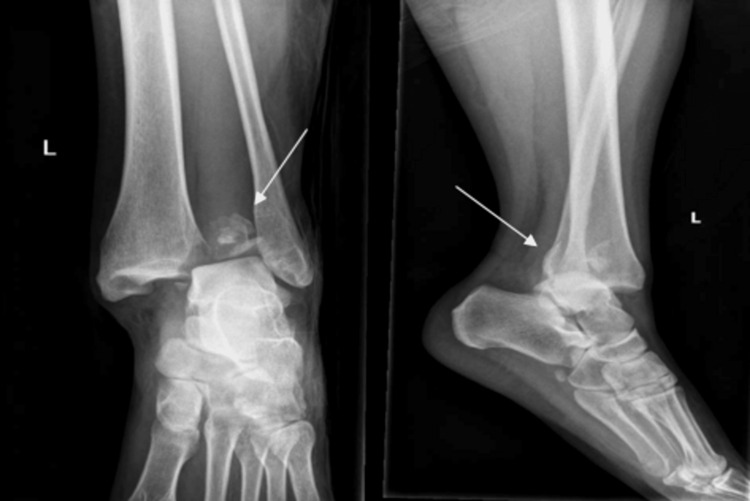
Left ankle anterioposterior/lateral X-ray images depicting a posterior malleolar fracture with displacement of the fragment into the syndesmotic gap
